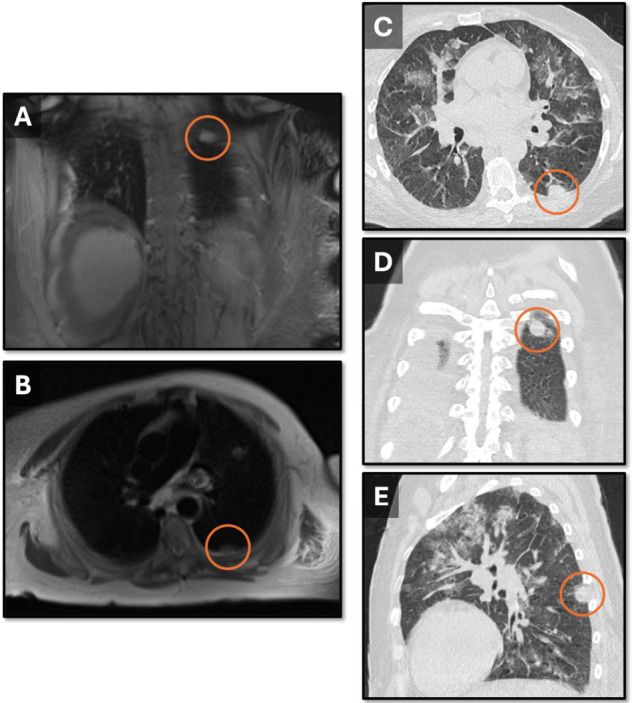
Left costal pleural nodule in a patient with a known primary of infiltrative hepatocellular carcinoma involving segment 8 of the liver with short segment tumor invasion of the proximal right portal vein. MR images at baseline in (A) coronal T1 3D Fat Saturated Post Contrast and (B) axial T2 Single-Shot Fast Spin Echo (SSFSE) and 5 days later on CT with (C) axial, (D) coronal, and (E) sagittal views. The red circle highlights the concerning pulmonary nodule. Nodule measures 14 mm in longest dimension at baseline and 5 days later. Nodule was suspicious for malignancy based on size.
